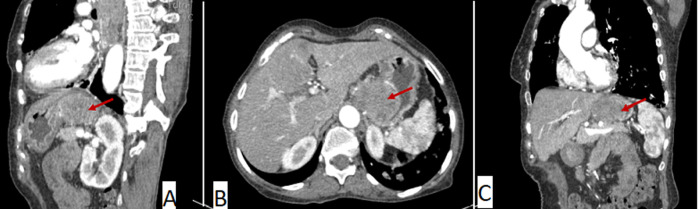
A) sagittal; B) axial; C) coronal images of the abdominal computed tomography scan showing the tissular stomach mass, heterogenous with exophytic development (red arrow)
